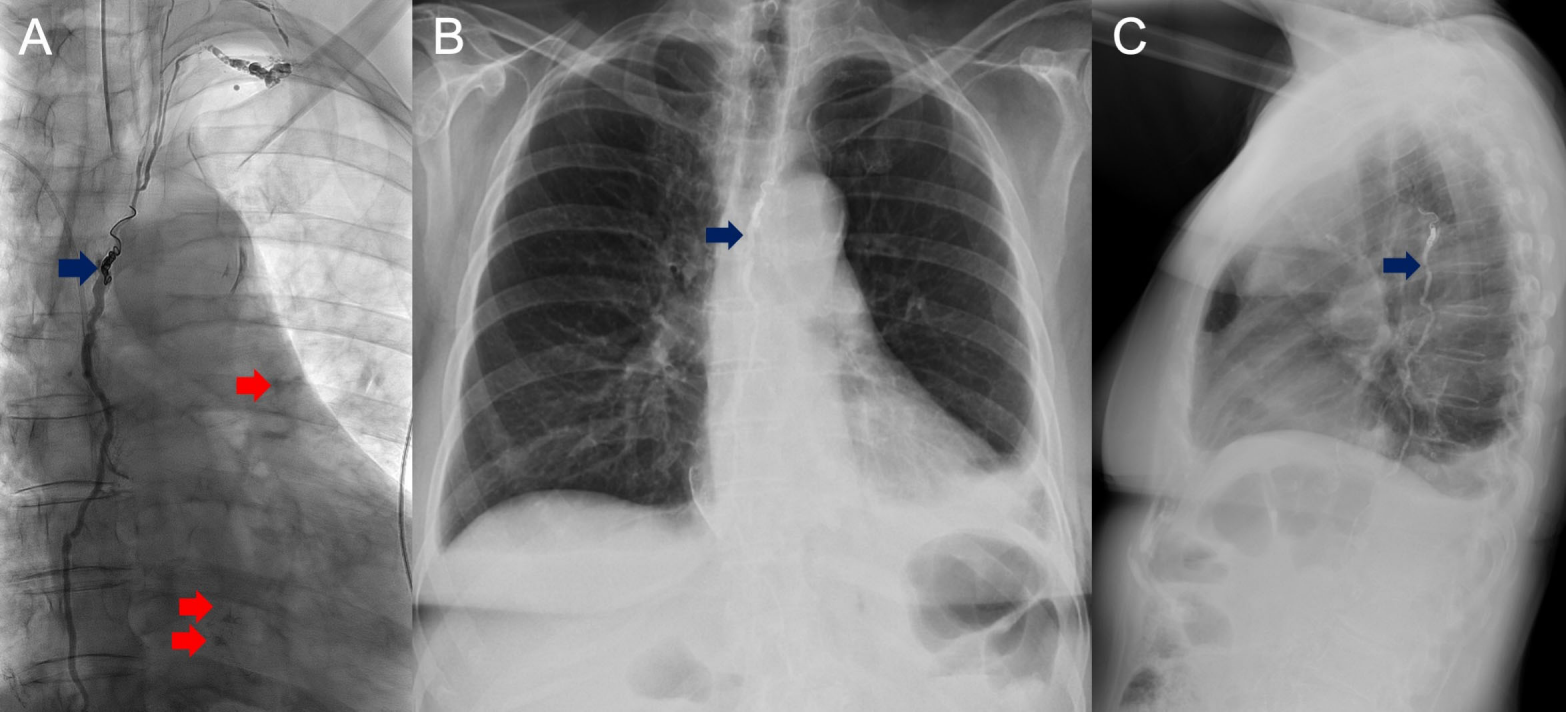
Anteroposterior (AP) orientation fluoroscopic images of Patient 1 with lipiodol-filled thoracic duct with areas of extravasation (red) visualized over the left lower hemithorax status post glue and coil embolization (blue) (A). Removal of left sided pigtail catheter at POD2, and AP (B) and lateral (C) chest radiograph obtained on POD7 demonstrating residual left sided effusion.
